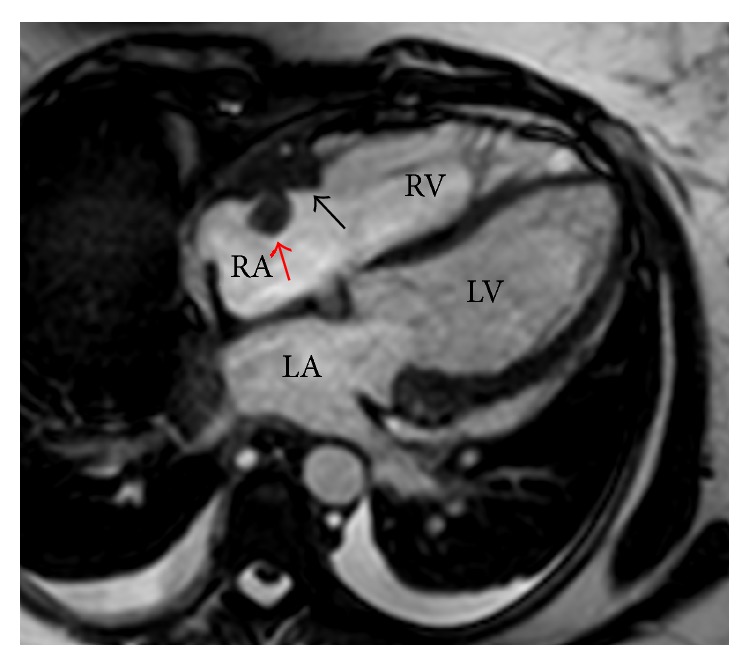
Steady-state free precession magnetic resonance image demonstrating a broad based, isointense structure (black arrow) measuring 3 cm × 1 cm arising from the lateral wall of the right atrium adjacent to the atrioventricular junction of the right atrium. Connected to this broad based mass is an isodense, circular, well-circumscribed mass (red arrow) measuring 1 cm in diameter protruding from the broad based mass into the right atrium. RA: right atrium, RV: right ventricle, LA: left atrium, LV: left ventricle.
